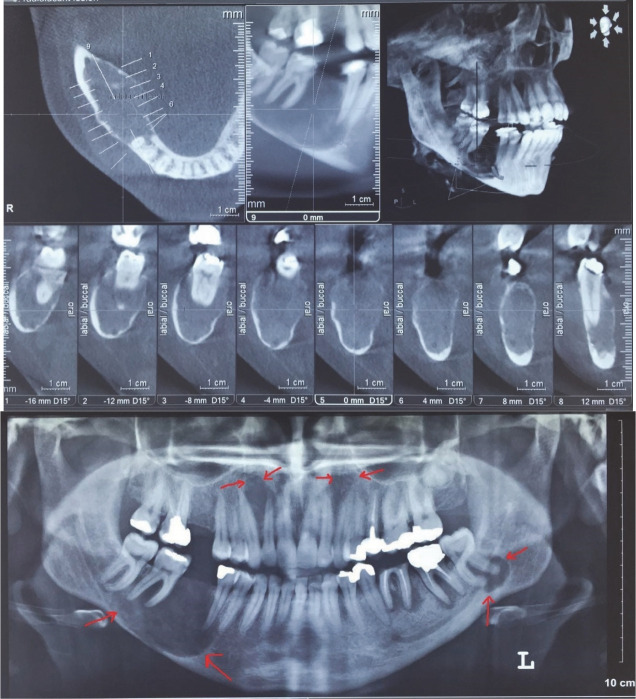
Pre-operative cone-beam computed tomography (CBCT) view, buccal and lingual bone thinning, and perforation, push the inferior alveolar nerve toward the lower border
